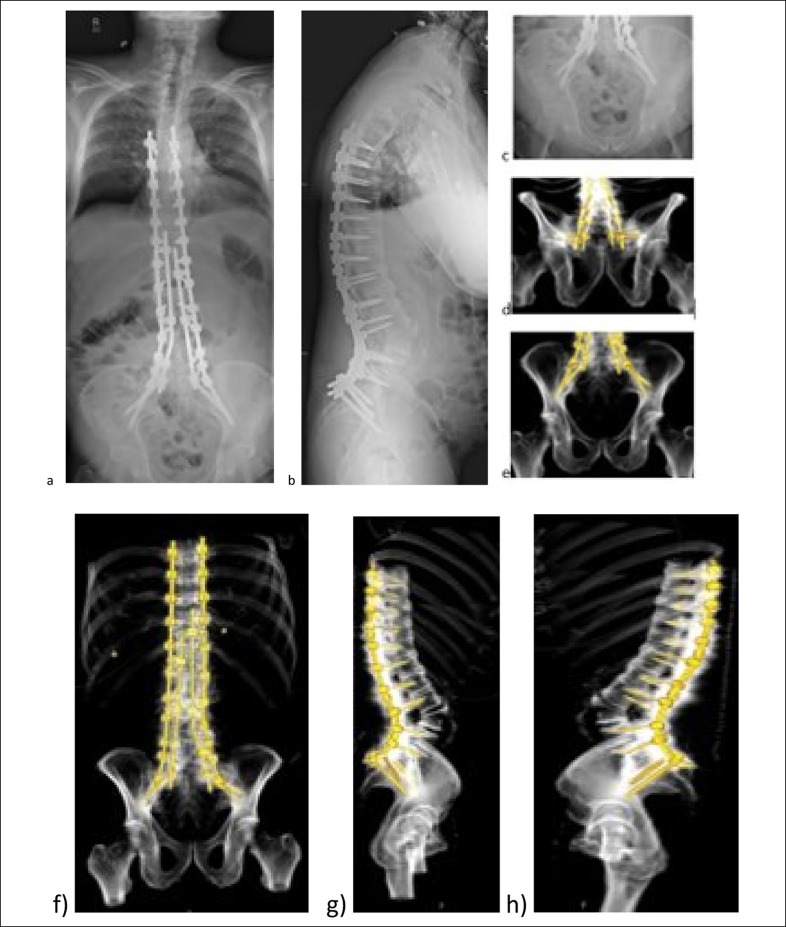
A, AP and (B) lateral standing radiograph showing restoration of the sagittal balance. C, Pelvic inlet view demonstrating the iliac screw trajectories within the iliac wings. D, Outlet and (E) AP 3D reconstruction from CT demonstrating the placement of the pelvic instrumentation. F, PA, (G) right, and (H) left lateral 3D reconstruction from CT demonstrating the placement of the quad-rod spinal instrumentation.
